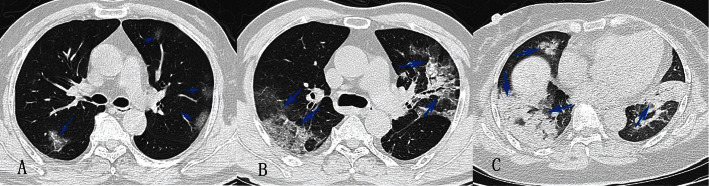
CT grading. (a) Mild pneumonia (peripheric and subpleural damping as ground glass). (b) Moderate pneumonia (thick shadow with multiple lung lobes (≥3) affected; cloudy flocculent or stone like lesions; local lobes consolidation (≥2); lobes fibrosis or sign of air bronchograms). (c) Severe pneumonia (consolidation with a minimum of 80% of the pulmonary or 4 lobes involvement; strip-shaped lesion and significant fibrosis).
